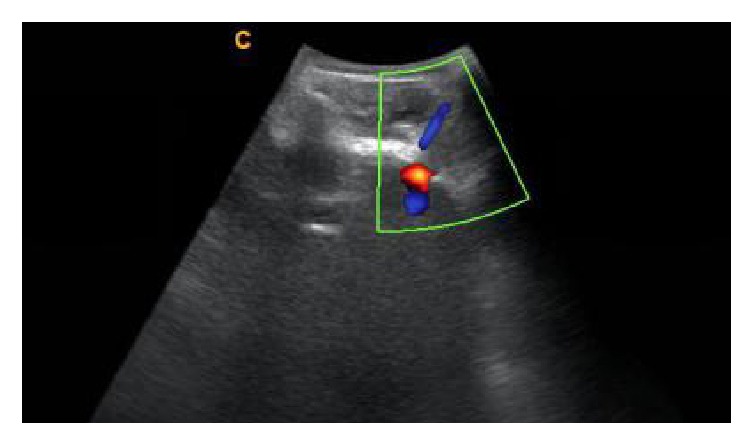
Transverse ultrasound image of the in-plane injection approach, the needle was inserted aiming to the Z-joint gap.
